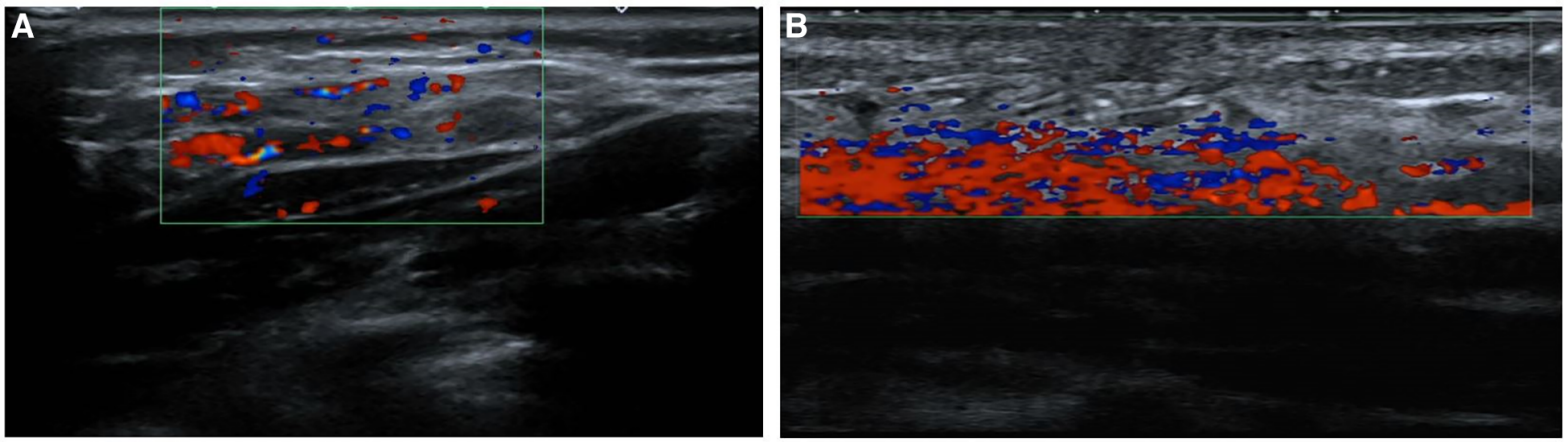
Ultrasound image of a patient with infantile hemangioma located at trunk, and was treated with oral propranolol. (A) Before treatment, the tumor body had abundant blood flow signals; (B) after 5 months of treatment, the blood flow signal inside the tumor had completely disappeared.
